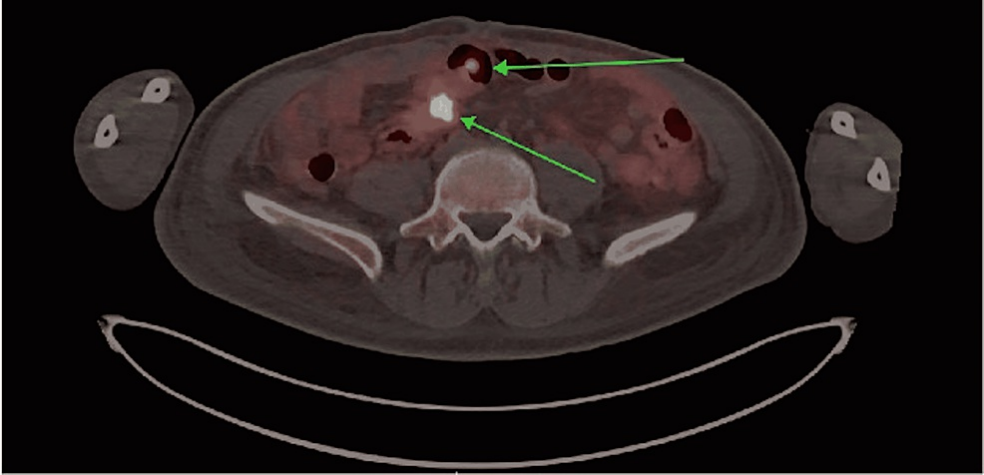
A PET/CT scan revealing a 3.3-cm focus (green arrows) of the upregulated somatostatin receptor in the small bowel consistent with a primary neuroendocrine tumor PET/CT, positron emission tomography/computed tomography
